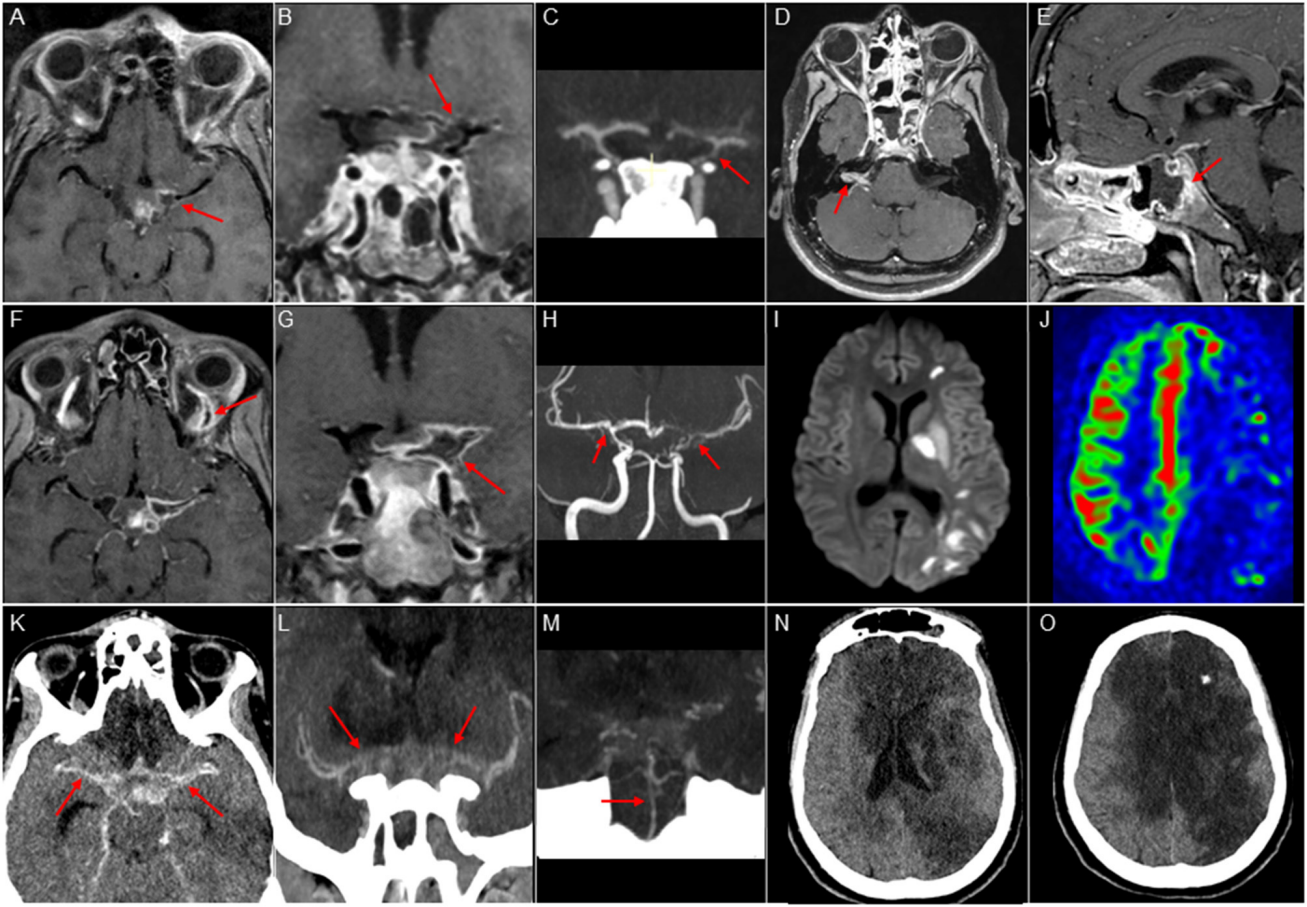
Imaging features. Upper panel: MRI and CT scan at admission. (A,B,D,E): Cube T1 FatSat post Gadolinium contrast, axial (A,D), coronal (B), and sagittal (E) views. (C): Contrast-enhanced CT with arterial phase, coronal view. Vasculitis of the Circle of Willis: stenosis of the left internal carotid artery, middle cerebral artery, and anterior cerebral artery (A-C), with vessel wall enhancement (A,B). Right acoustic-facial neuritis (D, arrow), sphenoid sinusitis with adjacent osteitis (E, arrow). Medium panel: MRI after sudden right hemiplegia and aphasia. (F,G) Cube T1 FatSat post Gadolinium contrast, axial view; (H) 3D Time-of-Flight angio-MR coronal view; (I) DWI sequence, axial view; (J) Arterial Spin Labeling perfusion sequence, axial view. Recent arterial ischemic stroke in the left middle cerebral territory (I) with extended hypoperfusion (J), worsening of severe and bilateral vasculitis (F-H), and left ophthalmic vein thrombosis (F, arrow). Lower panel: CT scan at day 14. (K): post-contrast axial view; (L,M) angio-CT coronal view; (N,O): axial view. Worsening of the intracranial vasculitis (K-M), with basilar artery involvement (M). Large bilateral infarcts in anterior and middle cerebral artery territories (N,O).
